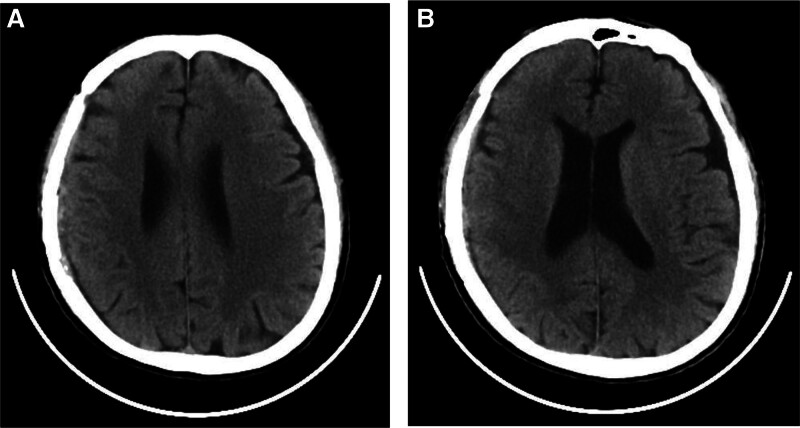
The results of the head CT scan of the patient 1-year post-surgery. (A) The low-density subdural mass in the right frontal, temporal, parietal, and occipital regions was resolved. (B) The centerline structure was centered.
